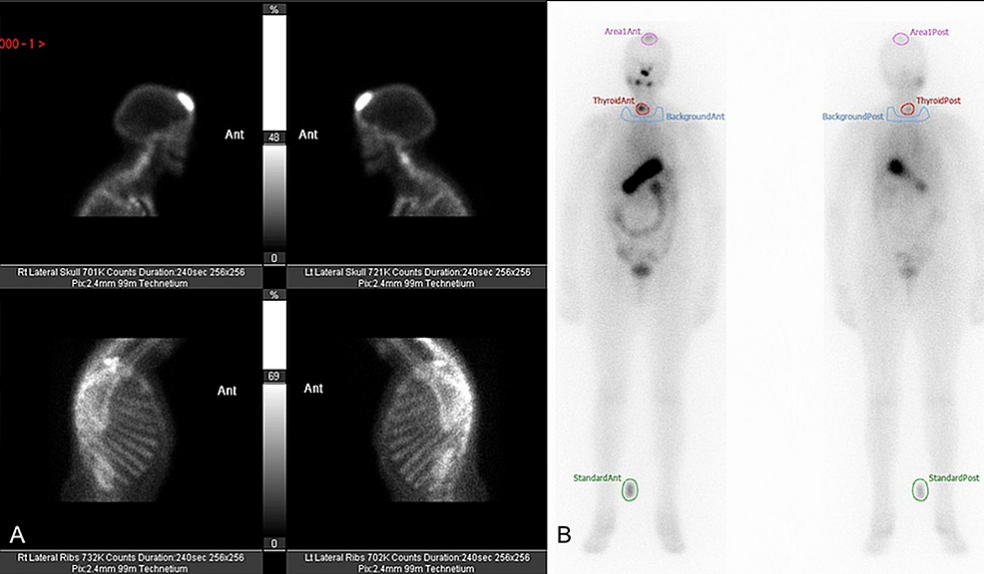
Nuclear medicine imaging showing areas of high uptake. (A) PET CT and (B) a nuclear medicine bone scan.
